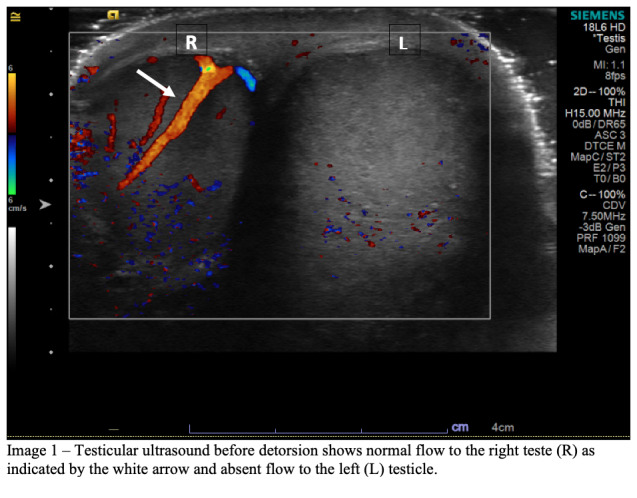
Testicular ultrasound before detorsion shows normal flow to the right testicle (R) as indicated by the white arrow and absent flow to the left (L) testicle.
